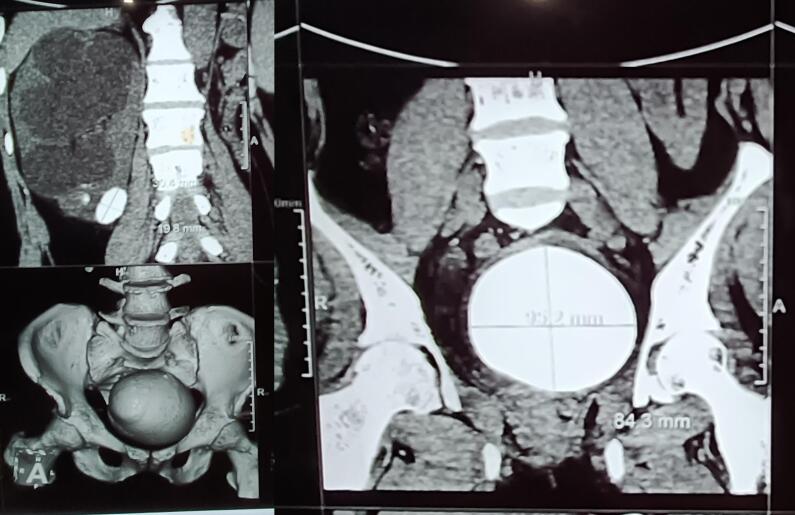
Plain CT of the kidneys, ureters, and bladder (KUB) showing Right Nephrolithiasis in lower poly calyx causing gross hydroureteronephrosis, left renal agenesis, and a large vesical calculus (10.6 cm × 8.6 cm × 8.8 cm, +1110 HU).
